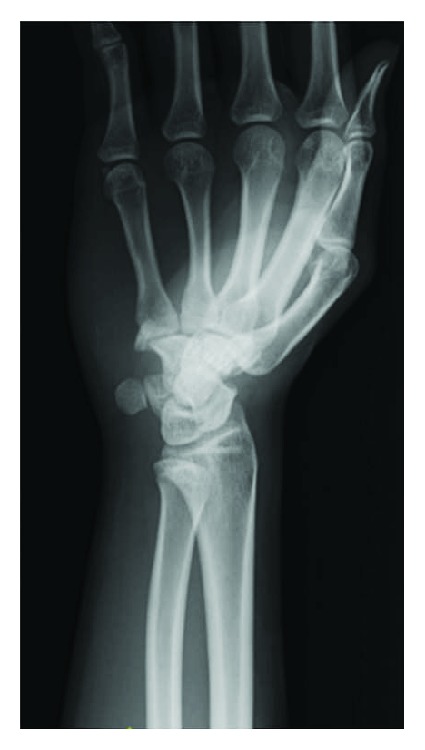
Radiograph taken at the previous clinic in supinated oblique position.
